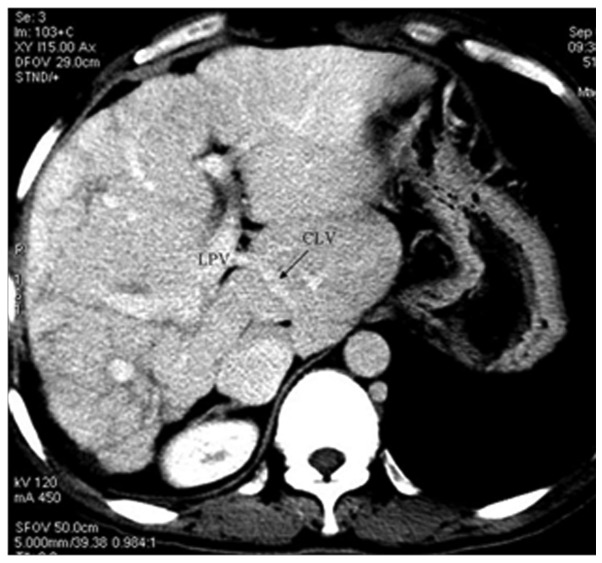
Portosystemic shunts. Computed tomography angiography shows the LPV connecting with the inferior vena cava via the patent CLV. LPV, left portal vein; CLV, caudate lobe vein.
